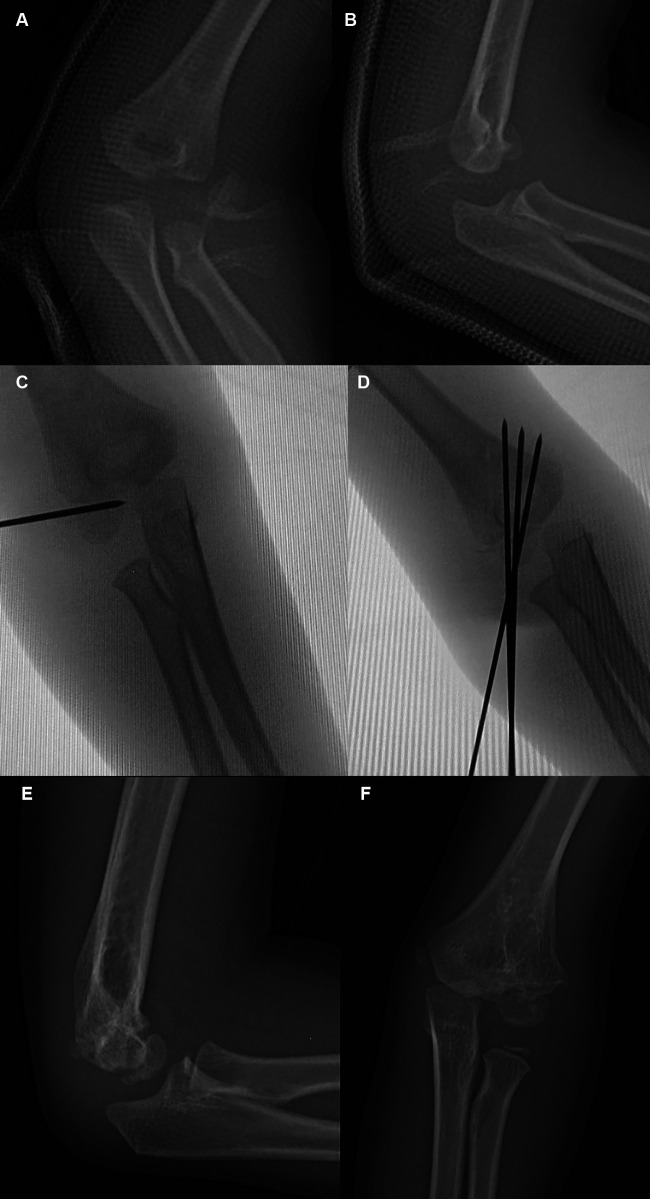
Typical x-ray of Type II degree I fractures. Anteroposterior (AP) (A) and lateral radiograph (B) showing a severe fracture with a single rotation of the distal fragment. The fracture is classified as a Type II degree I (unstable) fracture. A C-arm radiograph (C) showing leverage reduction with a joystick from the lateral side. (D) AP image showing almost satisfactory reduction of the fracture. Typical X-ray of Type II and Degree I. Lateral (E) and AP (F) radiographic images at 7 months postoperatively.
